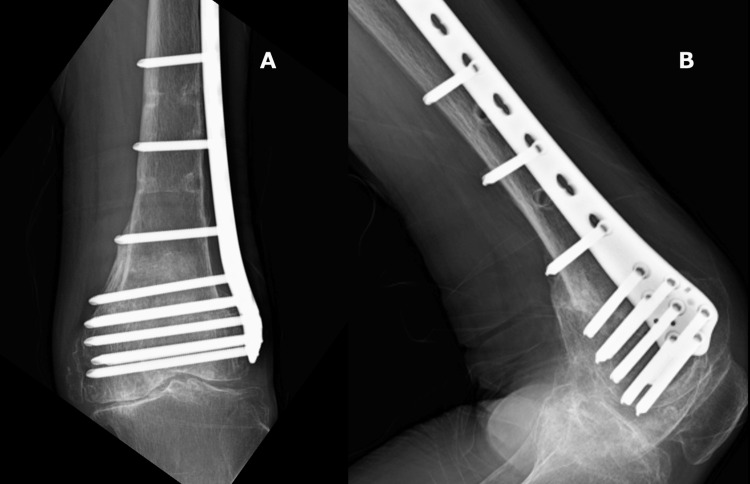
Postoperative radiographs at four-month follow-up A: anteroposterior view; B: lateral view
